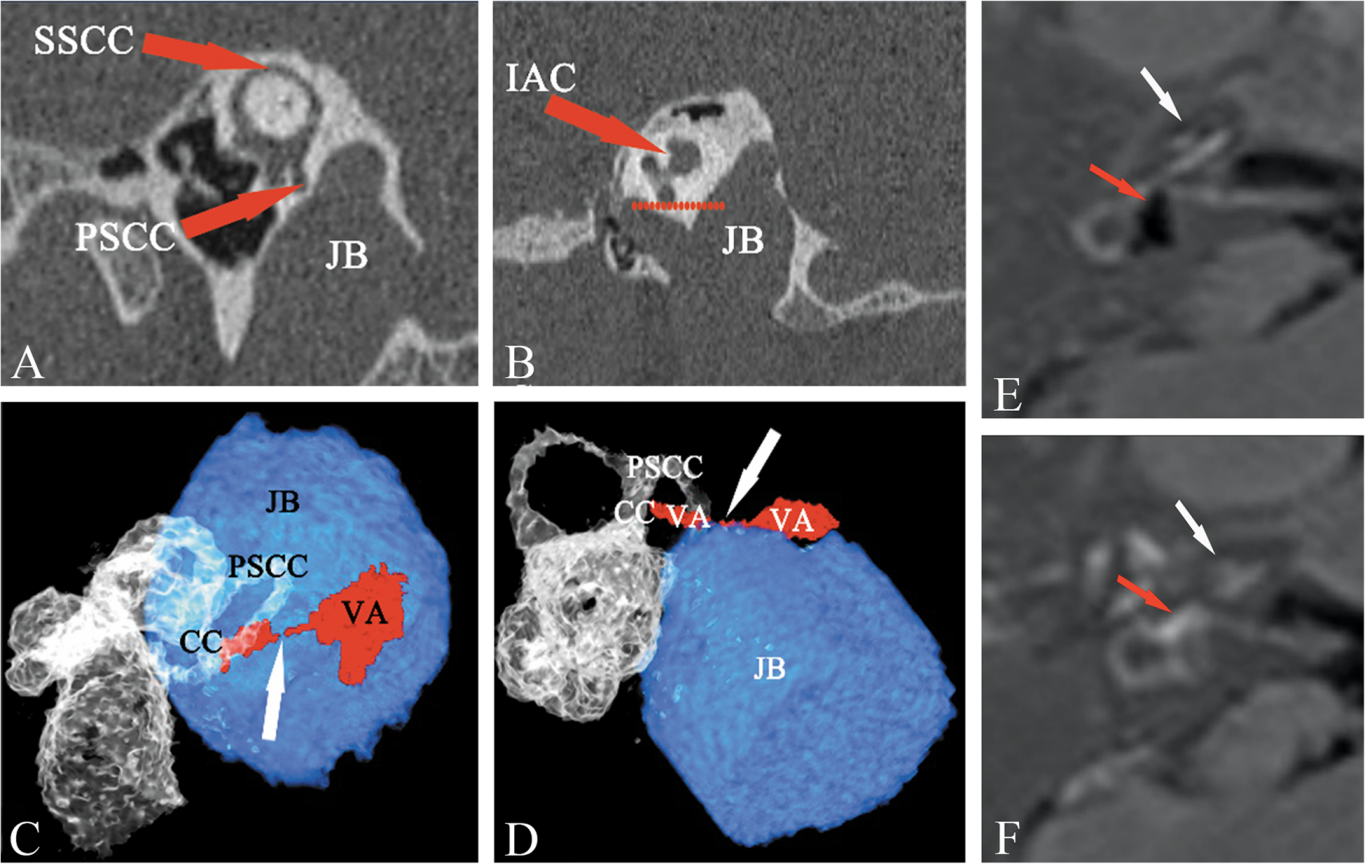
Type 4 JB in a hydropic ear. Type 4 was demonstrated in the (a) Pöschl and (b) sagittal planes with the JB well above the inferior margin of the PSCC, as well as the inferior margin of the IAC, which was imaged at the fundus with the modiolus. The external aperture of the VA was not visualized in the Pöschl plane (a) and sagittal plane (b), where an upward extension of the bulb invaded the region of the VA. c and d Three-dimensional reconstruction computed tomography showing the type III anatomic relationship between JB and VA, where VA obliteration (white arrow) was observed on the posterosuperior view (c) and an upward JB encroachment upon the VA resulting in VA obstruction (white arrow) was observed on the posteroinferior view (d). 3D-real IR MRI showed an extremely large EH in the vestibule (red arrow) with significant cochlear hydrops (white arrow) before surgery (e). Postoperative image showed the cochlear and vestibular hydrops disappeared, where normal bright perilymphatic fluid was visible in both cochlea (white arrow) and vestibule (red arrow) (f). CC, common crus; JB, jugular bulb; IAC, internal auditory canal; PSCC, posterior semicircular canal; VA, vestibular aqueduct; SSCC, superior semicircular canal; 3D-real IR, three-dimensional real inversion recovery
